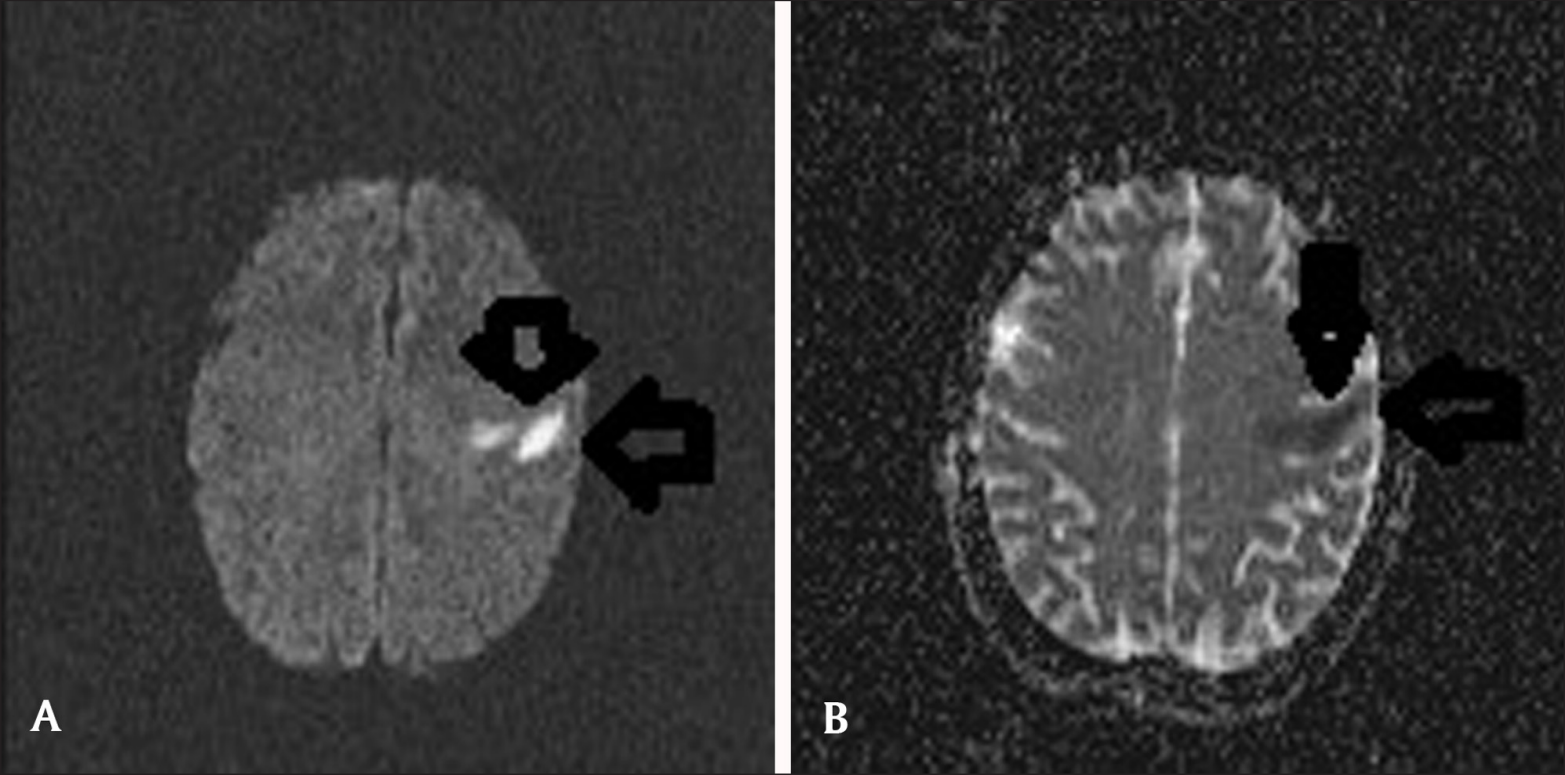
Admission bloods showed an elevated ESR and anaemia, while blood cultures unexpectedly grew Granulicatella adiacens. A transoesophageal echocardiogram showed vegetations involving the mitral and aortic valves. Both valves were repaired and postoperatively he complained of dyspnoea. A CT pulmonary angiogram was negative for pulmonary emboli but revealed multiple large splenic artery aneurysms, a clinically unexpected but significant complication of infective endocarditis (Figure 3A and 3B, black arrows). These were treated with an emergency splenectomy. Based on these imaging finding this was a case on infective endocarditis with extra cardiac complications causing both an embolic stroke and mycotic splenic artery aneurysms.
